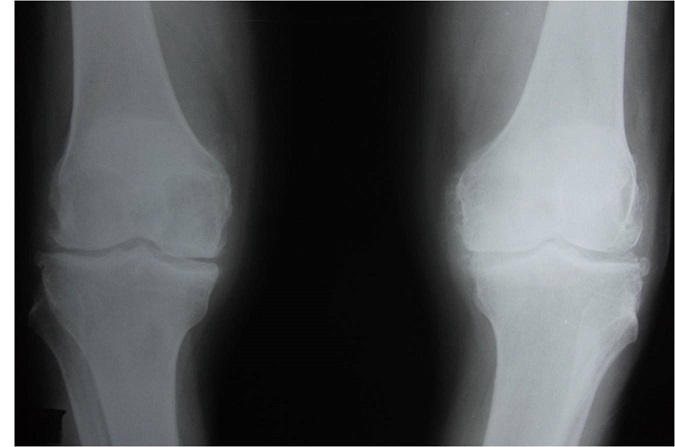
Radiographie des genoux: aspect de chondrocalcinose articulaire
